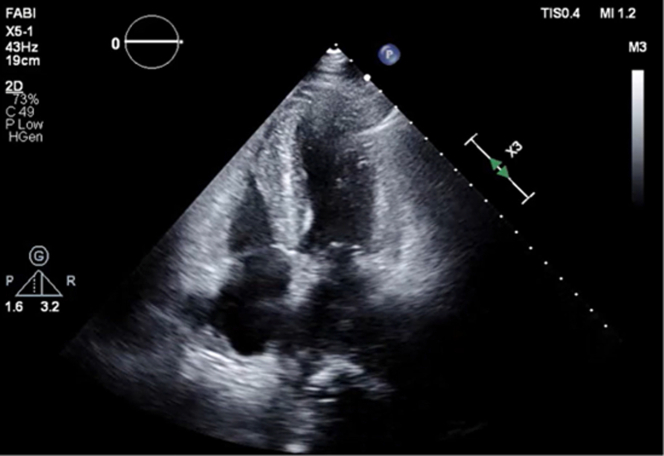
Repeat Transthoracic Echocardiogram Findings included severely reduced left ventricular ejection fraction of 25% and biventricular failure, small pericardial effusion, pacing wires, and left ventricular assist device.
